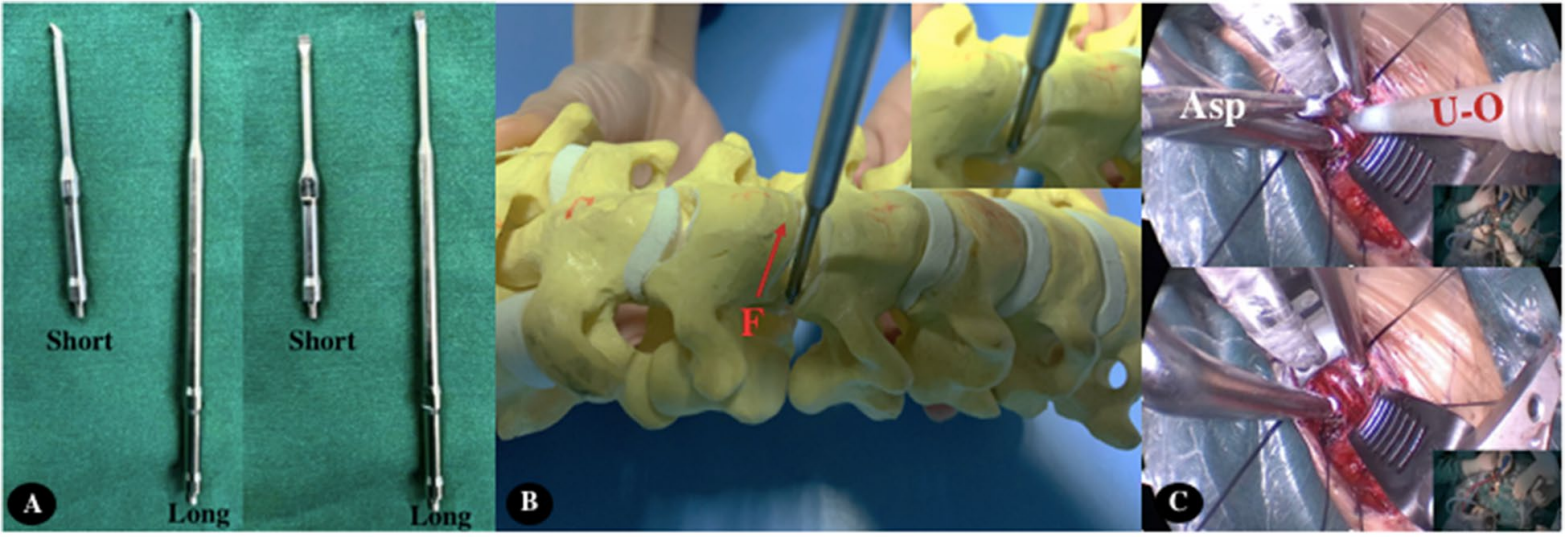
F stands for Force. A stands for Aspirator. U-O stands for ultrasonic osteotome. a represents Ultrasonic bone osteotome with spoon shaped tip. b is a model figure. The red arrow in the figure shows that the ultrasonic osteotome applies force to the dorsal side of the nerve. c shows the intraoperative image. The operator uses the ultrasonic osteotome with one hand, the auxiliary hand uses the aspirator, and both hands operate simultaneously to improve the surgical efficiency
